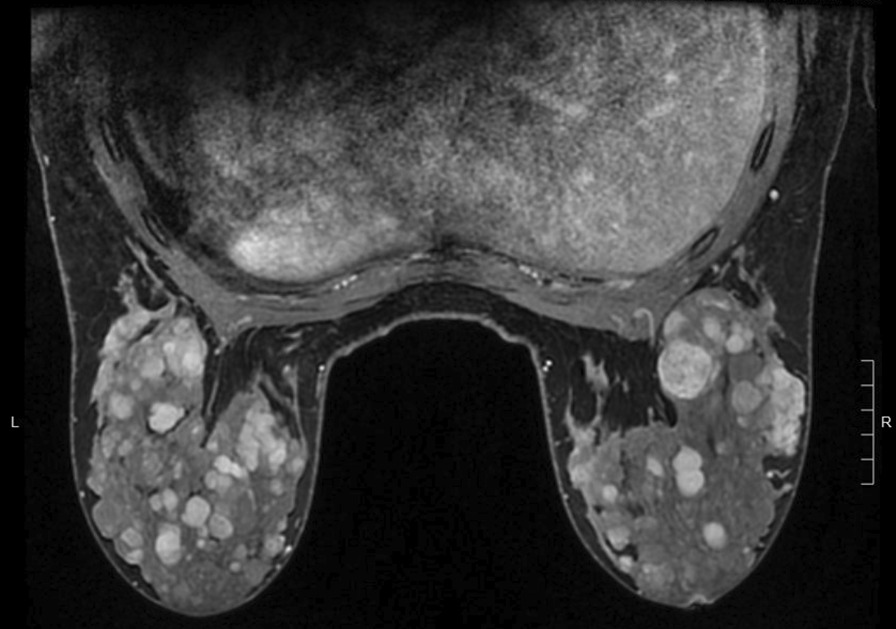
Contrast enhanced breast MRI shows innumerable bilateral breast masses ranging from 0.5 to 5 cm in size
